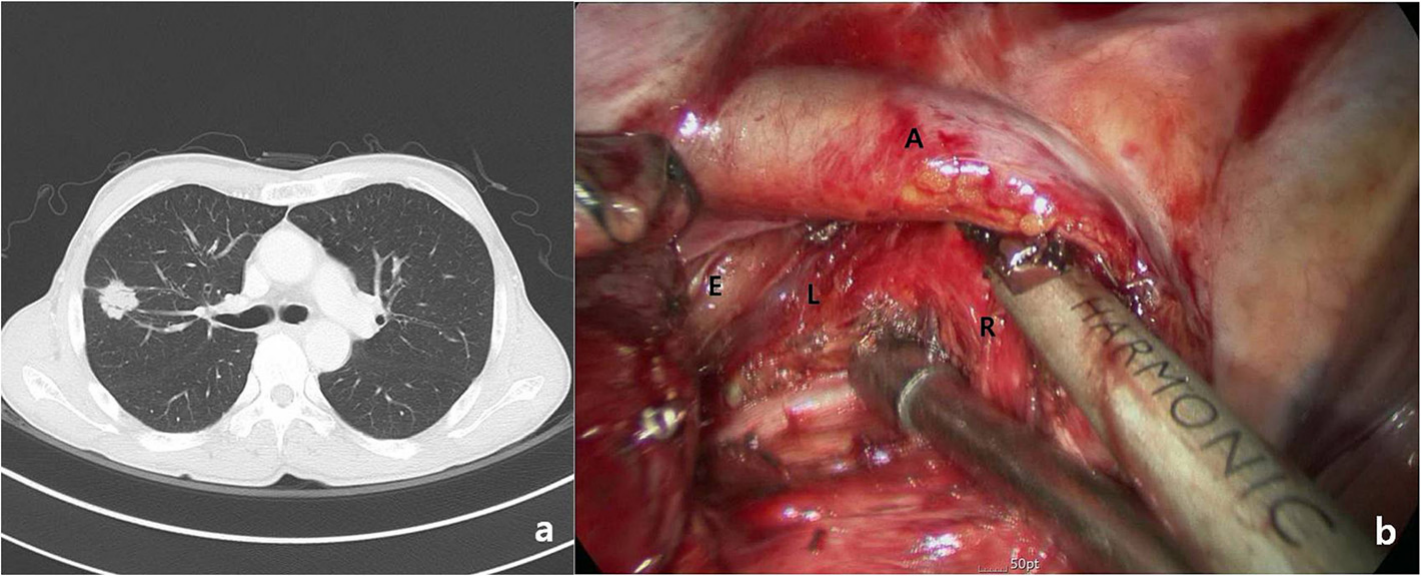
Chest computed tomography revealed 1.8 cm nodule with spiculation in Right upper lobe (a) Subcarinal lymph node dissection (b) bounded by right main bronchus (R) and left main bronchus (L). A: Ayzgos vein, E: Esophagus.
